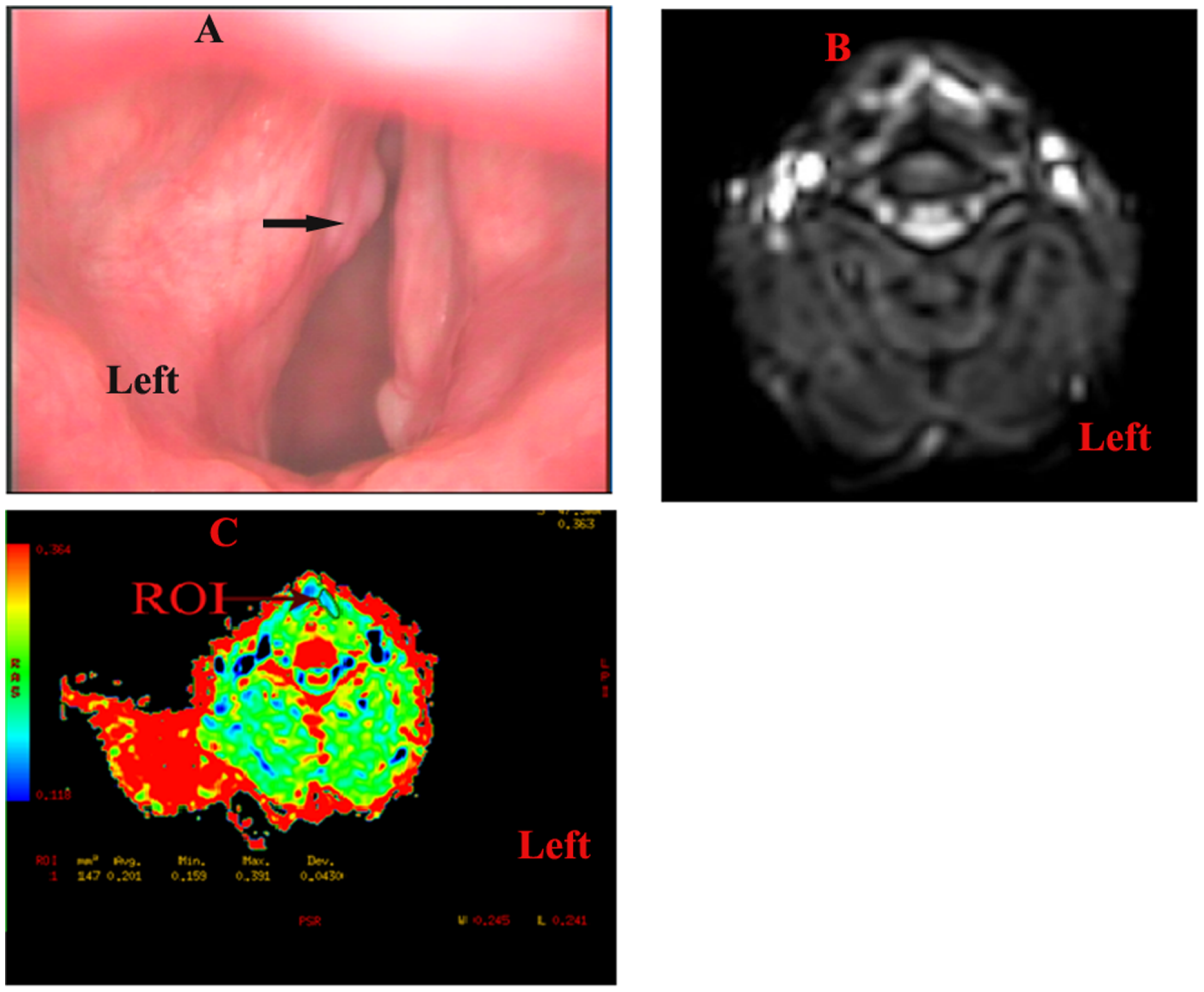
A patient with a pathologically proven laryngeal precancerous lesion. A: A lesion in the left vocal cord was suspected to be laryngeal carcinoma by laryngostroboscopy. B: DWI suggested hyperintense lesions in the left vocal cords (b = 1000 s/mm^2^). C: The corresponding ADC map also revealed hyperintense lesions in the left cord (ADC = 2.01×10^−3^ mm^2^/s). These findings suggest that the lesion in the left vocal cord may be benign.
